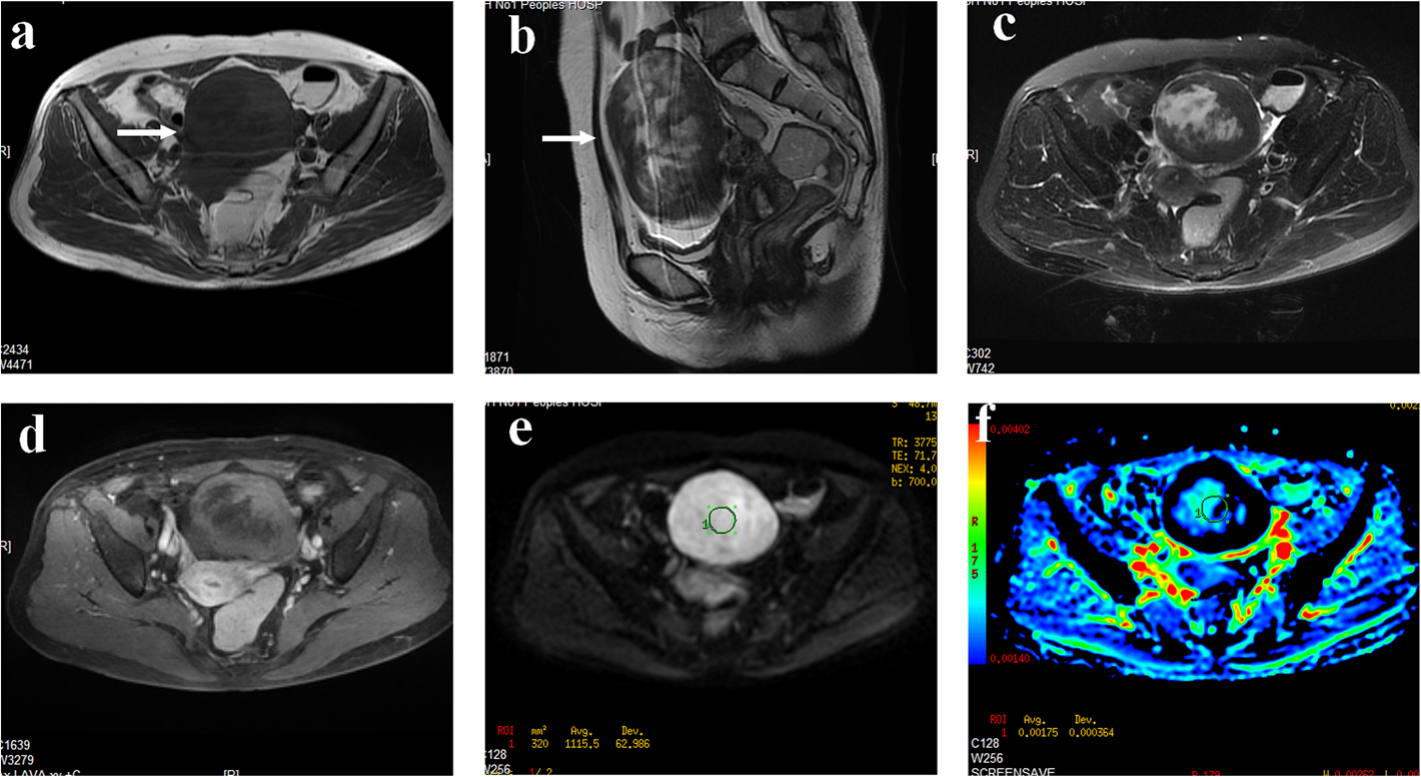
A 57-year-old female patient with thecoma. (a) Axial T_1_WI revealed a solid mass with a mostly iso–hypointense signal in the left adnexal region (arrow). (b) On sagittal T_2_WI, the solid tumor, 116 mm in maximum diameter, showed a heterogeneous signal with patchy high signal located eccentrically and an intermediate signal located peripherally. (c) On fat-suppressed T_2_WI, the signal of the mass was similar to that in (b). (d) On contrast-enhanced fat-suppressed T_1_WI, the lesion showed mild enhancement, less than that of the myometrium. (e) On DWI-MRI (*b* = 700 s/mm^2^), the lesion appeared as a homogeneous hyperintense signal. (f) ADC map shows the hypointense signal with a marked peripheral hypointense signal. The ADC value at the corresponding site is 1.75 × 10^–3^ mm^2^/s.
